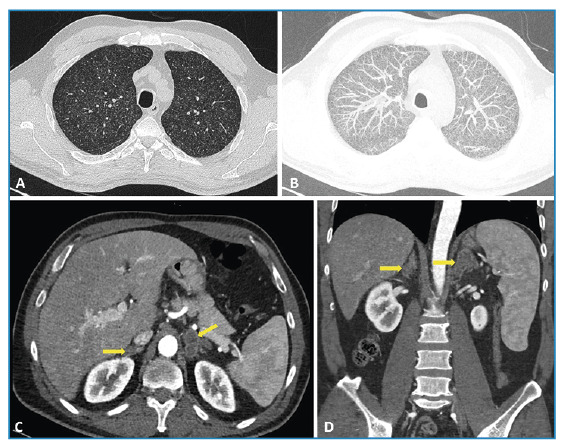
(A) Axial high-resolution computed tomography image showing numerous randomly distributed pulmonary micronodules. (B) Maximum intensity projection image demonstrating a diffuse miliary pattern throughout both lungs, consistent with miliary tuberculosis. (C) Axial contrast-enhanced abdominal computed tomography showing bilateral adrenal gland enlargement with hypodense, mass-like thickening (arrows). (D) Coronal reformatted image confirming symmetric bilateral adrenal involvement (arrows).
